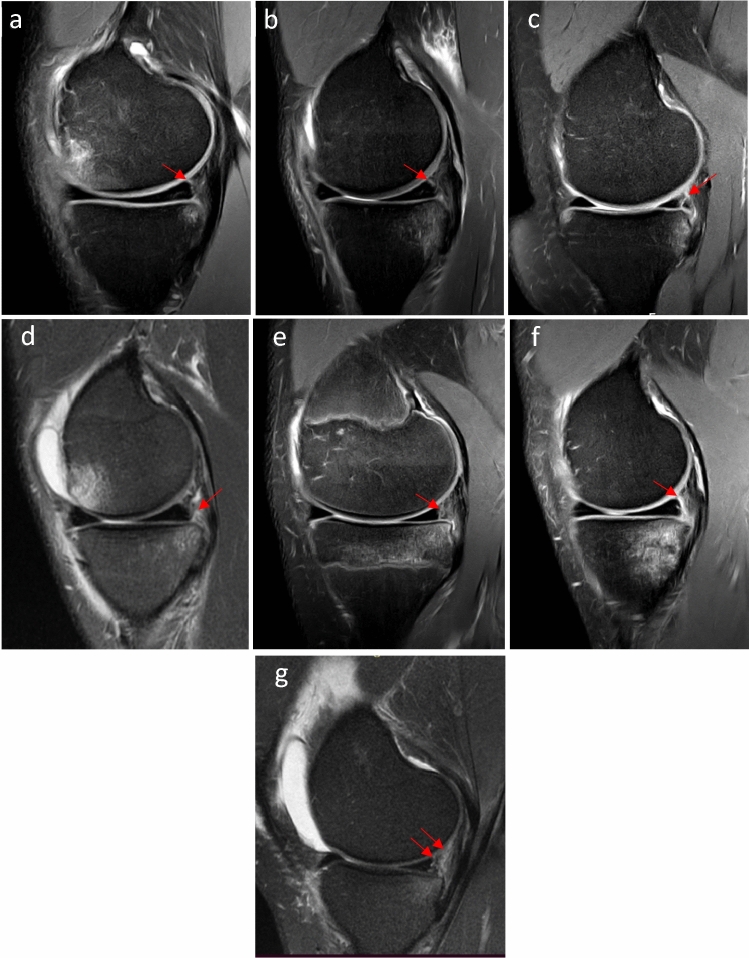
Meniscal ramp lesion subtypes (red arrow) on sagittal proton density fat saturation MRI sequences. a Type 1; meniscocapsular ligament tear, as shown by the linear vertical fluid signal reaching the superior articular surface. b Type 2; partial superior peripheral meniscal horn tear, as shown by the linear vertical fluid signal reaching the superior articular surface. c Type 3A; partial inferior peripheral posterior horn meniscal tear, as shown by the linear oblique fluid signal reaching the inferior articular surface. d Type 3B; meniscotibial ligament tear, as shown by the disruption of the ligament with a fluid signal. e Type 4A; complete peripheral posterior horn meniscal tear, as shown by the fluid signal extending from the superior to the inferior articular surface. f Type 4B; complete meniscocapsular junction tear, as shown by the fluid intensity signal extending from the superior to the inferior articular surface. g Type 5; peripheral posterior horn meniscal double tear, as shown by two parallel linear fluid signals extending from the superior to the inferior articular surface. Note that PMT bone bruising is present in all images. MFC bone brusining is present in a and d. *MFC* medial femoral condyle, *MRI* magnetic resonance imaging, *PMT* posteromedial tibial
